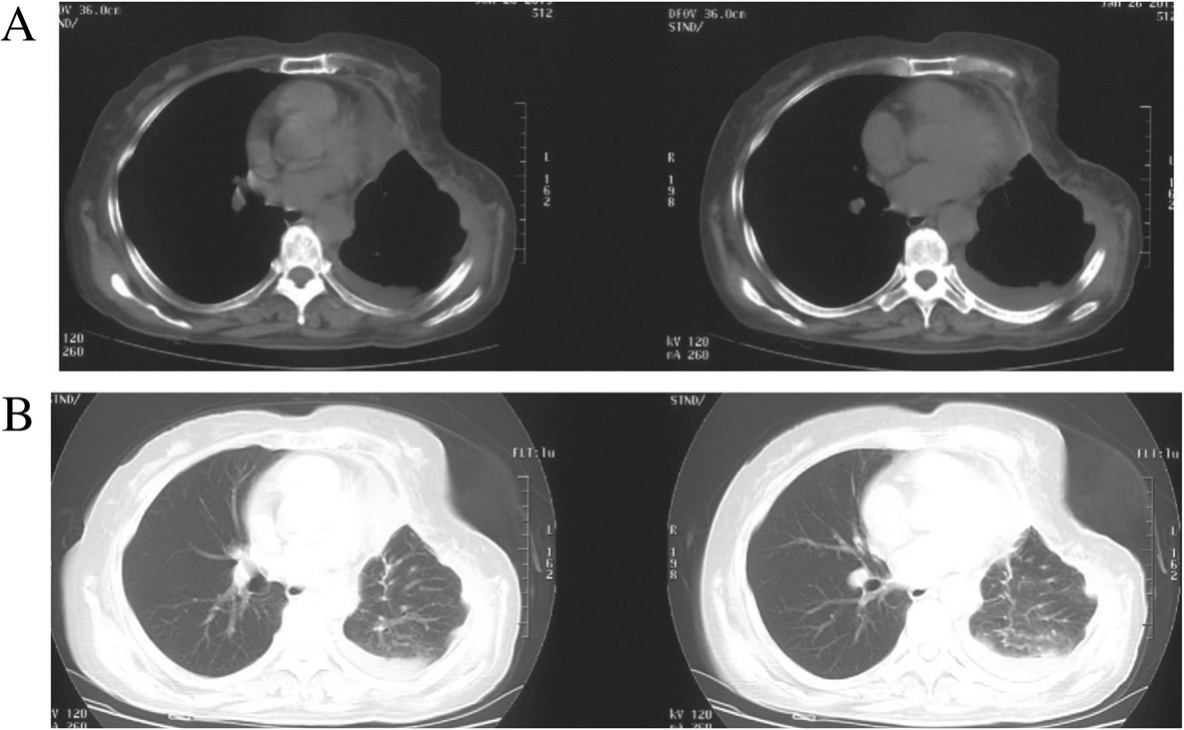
CT scans after operation. The chest CT scan was performed in 7 days after the operation. A: In the mediastinal window; B: In the lung window.
